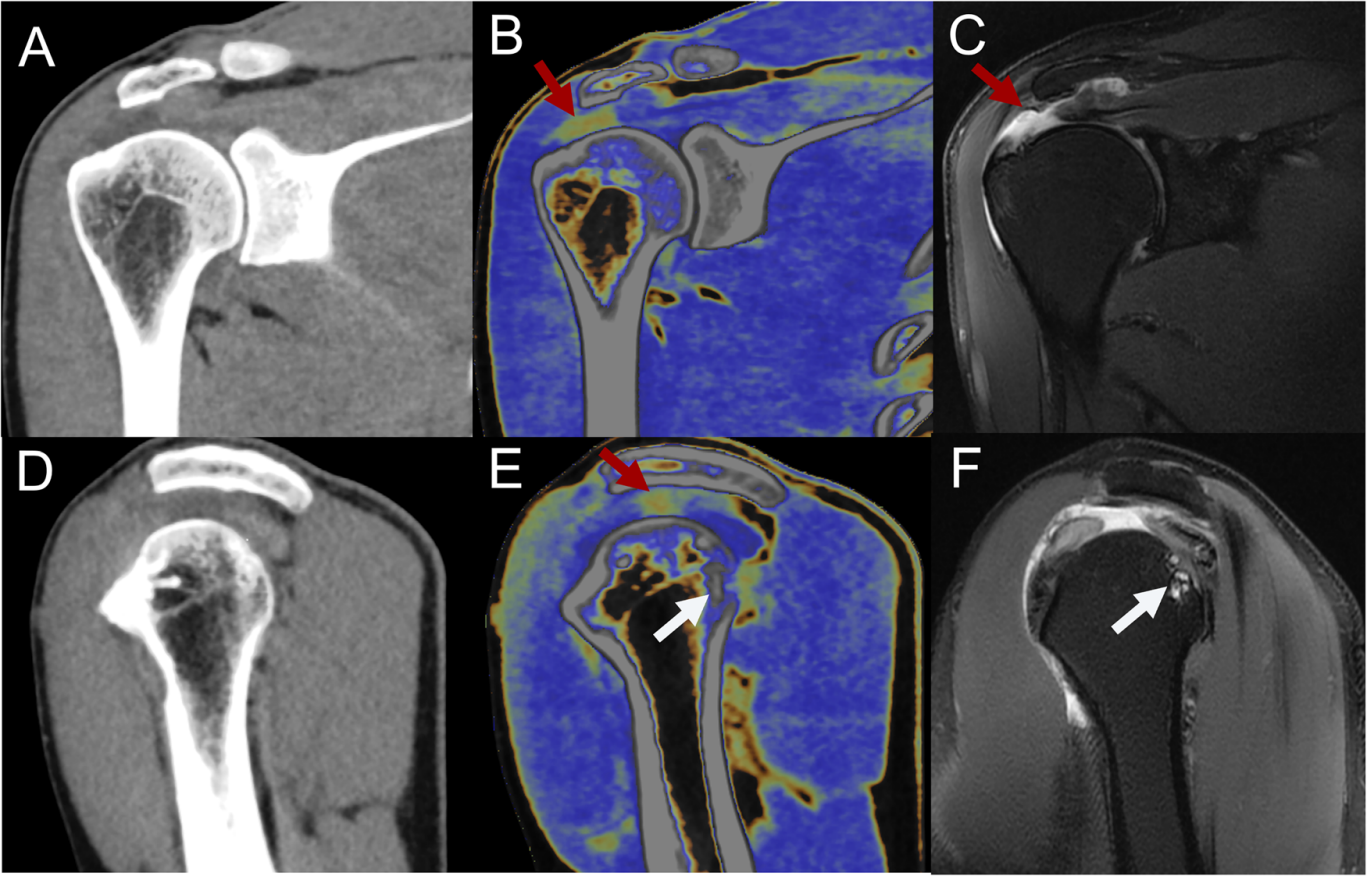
Images for a 56-year-old man presenting with acute severe right shoulder pain and swelling after exercise. A, D At standard CT, the supraspinatus tendon tear initially did not clearly show upon gray-scale reconstruction. B, E Supraspinatus tendon tears were detected by all readers after MMD reconstruction images were optimized for tendon analysis. C, F Noncontrast MRI in the oblique coronal and sagittal positions shows the supraspinatus tendon tear on fat-suppressed proton density-weighted imaging (red arrow). E, F Osteocortical discontinuity at the greater tuberosity of the humerus and subcortical cyst formation (white arrow). MMD, multi-material decomposition
